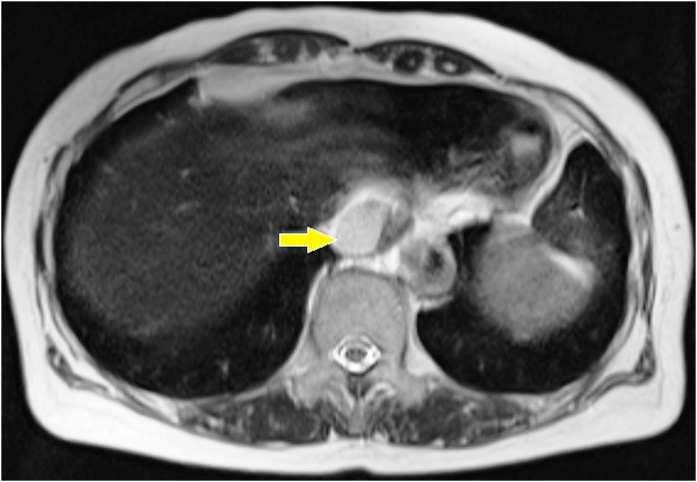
Magnetic resonance imaging findings. T2-weighted image showing a cystic mass with high signal intensity in the lower thoracic esophagus (arrow).
